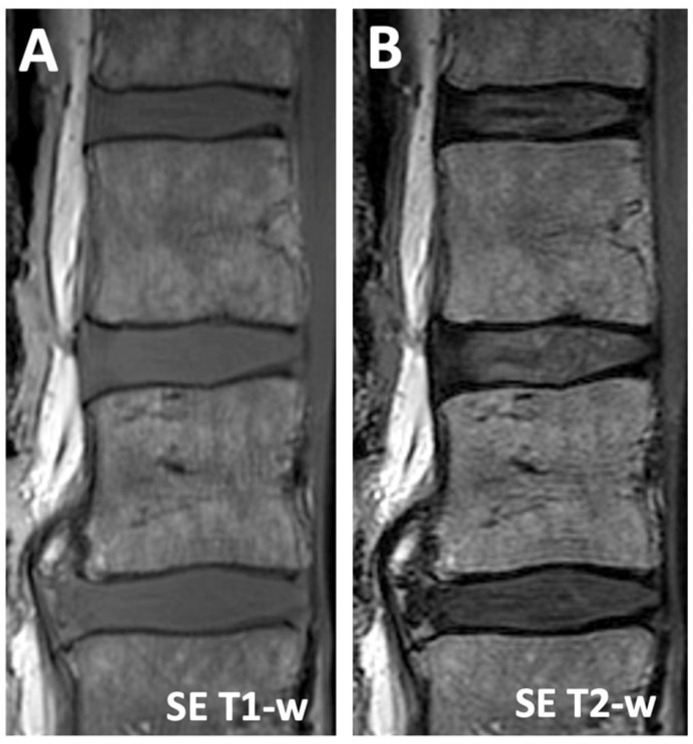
Conventional sagittal MR imaging of the human lumbar spine performed with (A) spin echo T1-weighted and (B) spin echo T2-weighted sequences. The cartilage endplate at the disco-vertebral junction (interface between vertebral body and intervertebral disc) has low signal intensity and is indistinguishable from the vertebral endplate, the cortical bone of the vertebral body.
